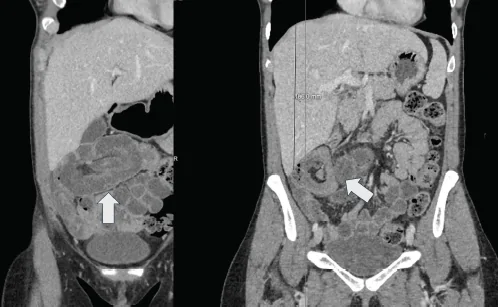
Contrast-enhanced computed tomography abdomen and pelvis (coronal reformatted images) demonstrating ileocolic intussusception (arrows) with characteristic “bowel-within-bowel” configuration. Mesenteric fat and vessels were dragged into the intussusceptum, producing a layered appearance consistent with the target sign. Findings indicate early small-bowel obstruction without pneumatosis or perforation.
